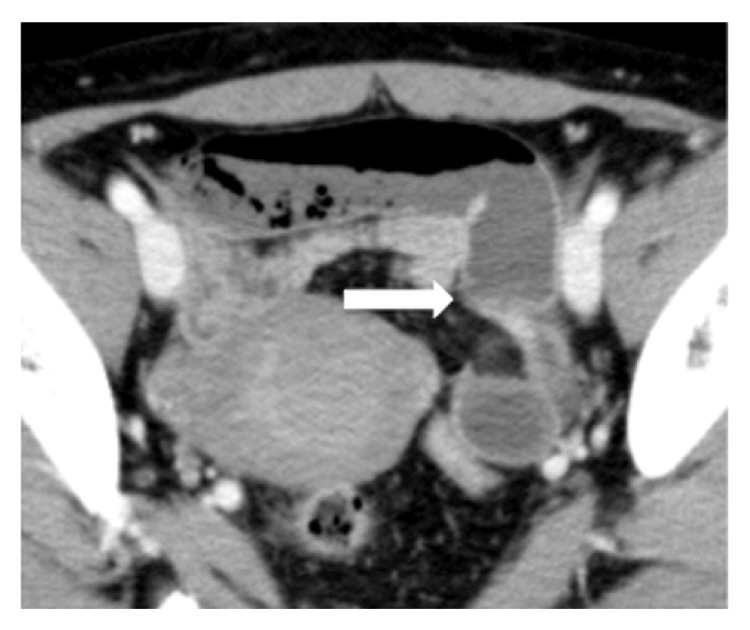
Abdominal computed tomography scan with intravenous contrast consistent with small bowel obstruction (axial view).* White arrow* shows the site of caliber change of the small intestine.
